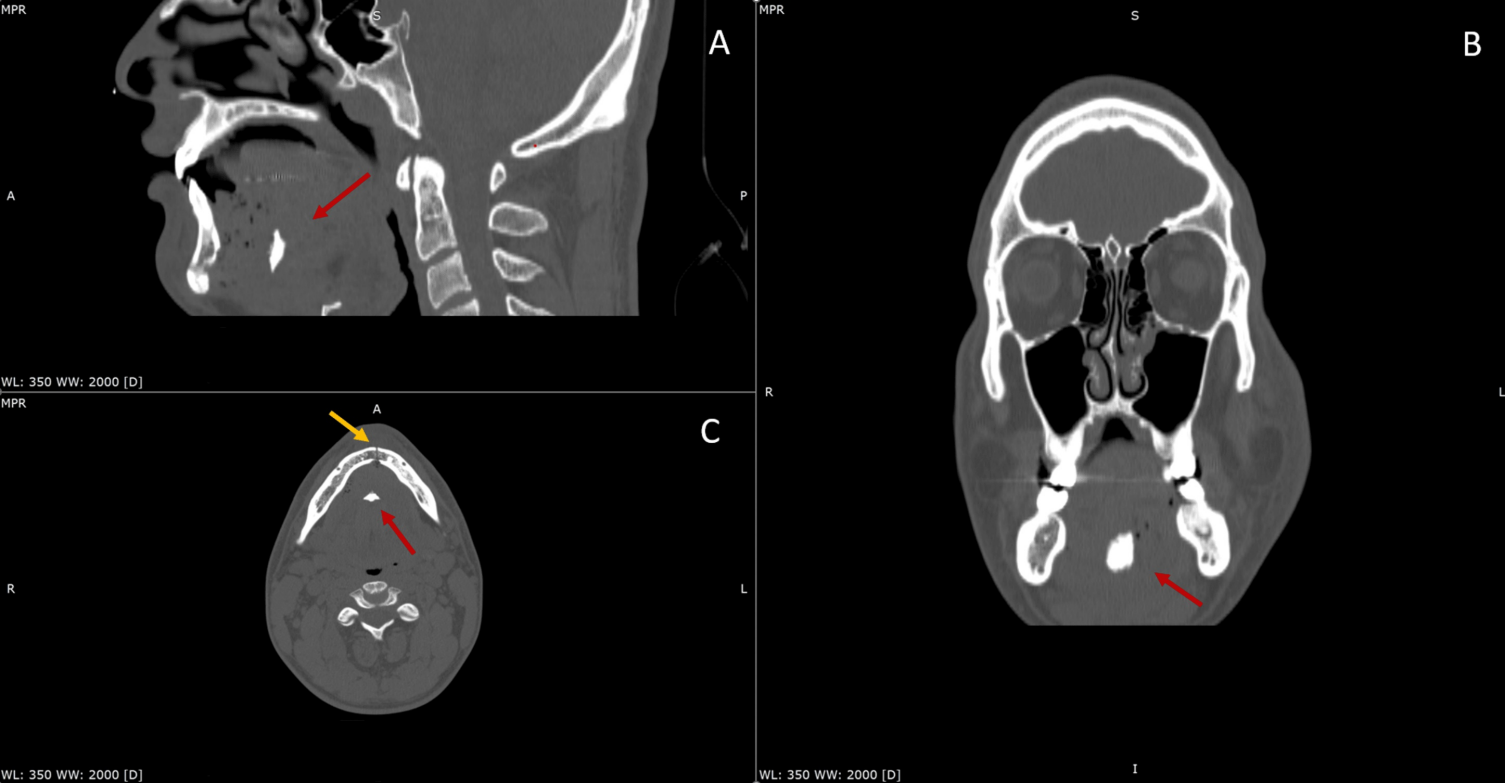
Multiplanar CT imaging of the mandible demonstrating the avulsive genial tubercle fracture and associated anterior mandibular injury. (A) Sagittal CT section showing the avulsed genial tubercle fragment (red arrow) displaced posteriorly toward the floor of the mouth. (B) Coronal CT section confirming the inferior and posterior displacement of the genial tubercle fragment (red arrow) in relation to the mandibular symphysis. (C) Axial CT section demonstrating the avulsive genial tubercle fragment (red arrow) and the midline mandibular fracture at the symphyseal region (yellow arrow), with associated sublingual emphysema.
